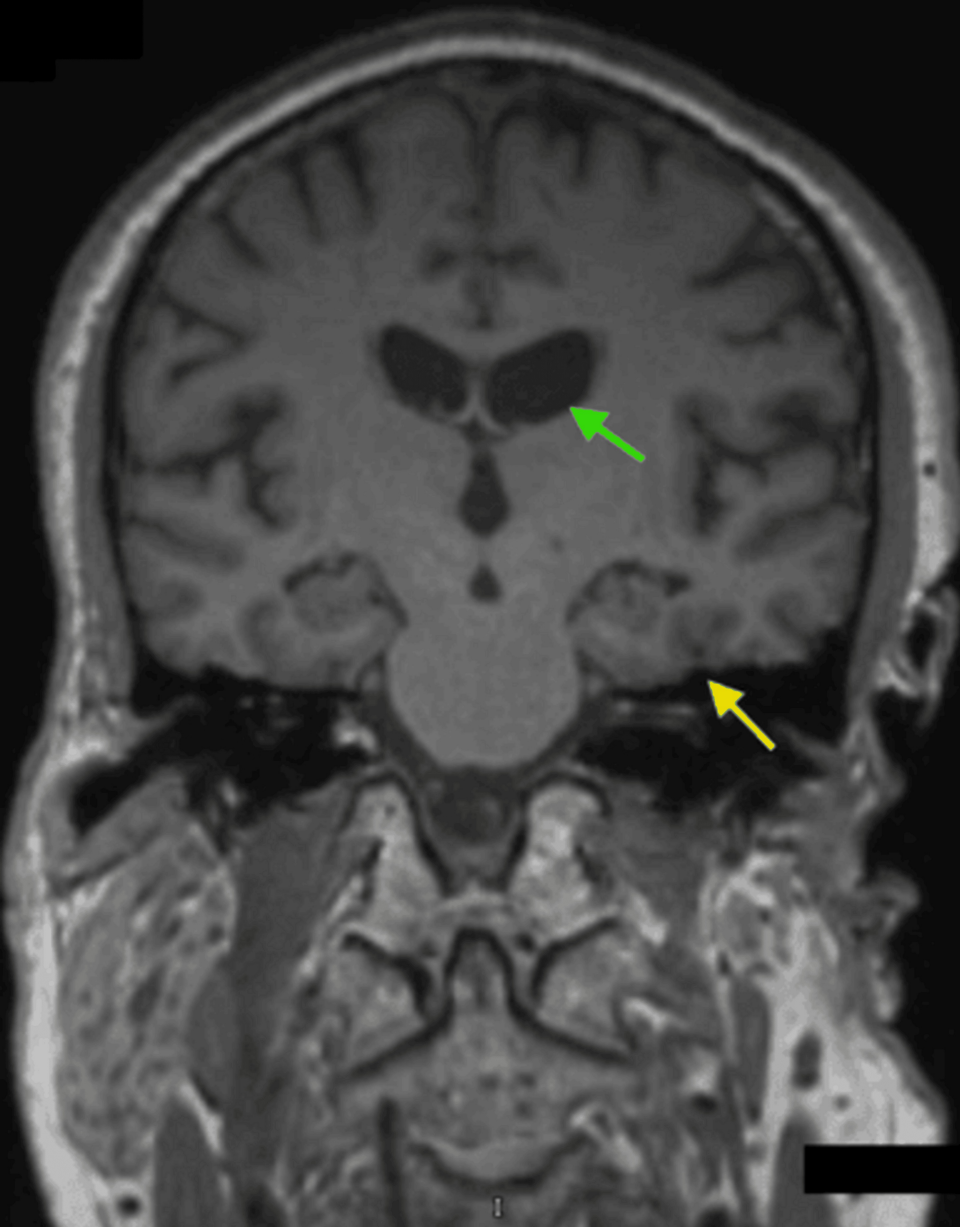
MRI of the brain demonstrating temporal lobe atrophy The green arrow is highlighting ventricular enlargement secondary to temporal lobe atrophy, which is highlighted by the yellow arrow.
